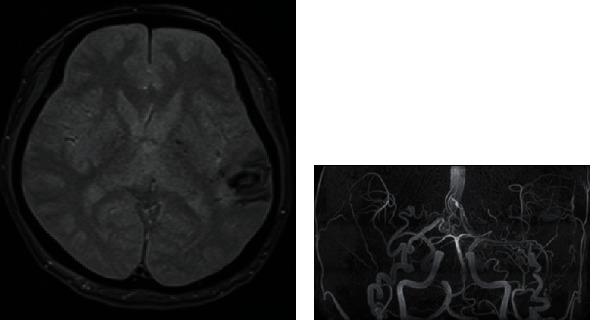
(a) T2 star-weighted magnetic resonance imaging revealed a hemorrhagic lesion in his left temporal lobe. (b) Magnetic resonance angiography revealed abnormal sinus signals.
